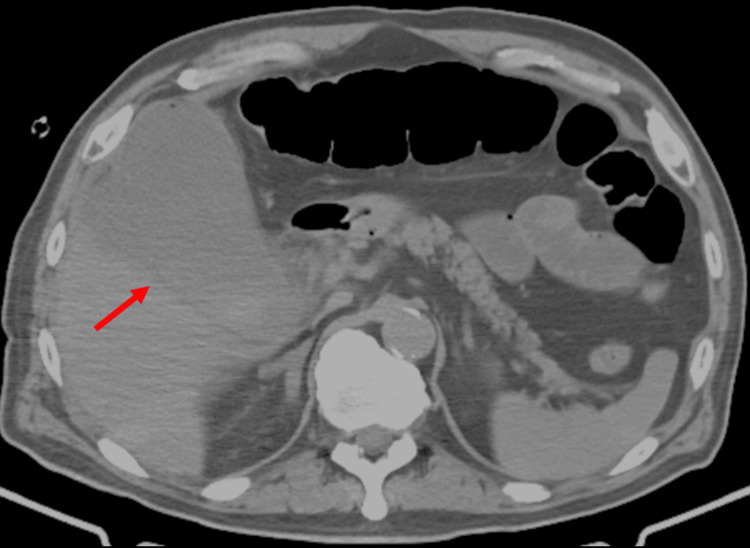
Unenhanced abdominal CT shows a gallbladder lesions. The red arrow points to an enlarged gallbladder. An abscess is noted on the liver surface, contiguous with the gallbladder.
